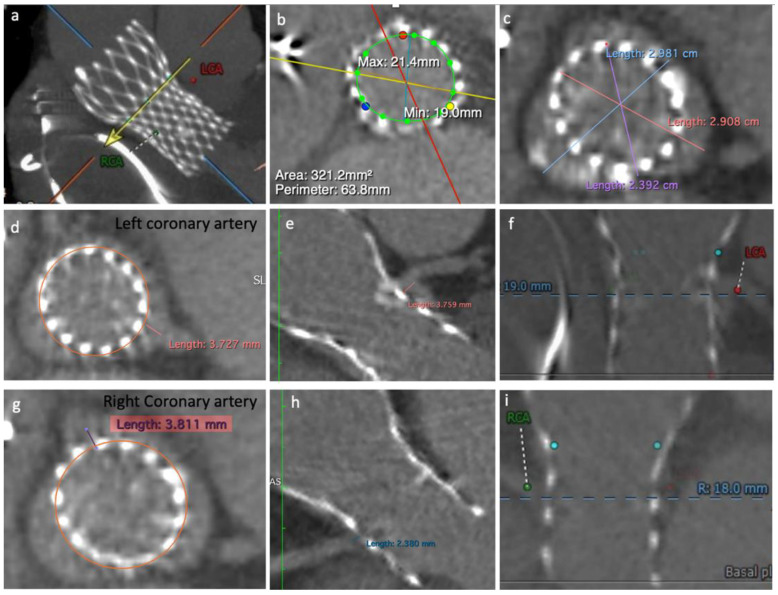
Computed tomography angiography images: (a) CoreValve structure, left coronary artery ostium (LCA) and right coronary artery ostium (RCA); (b) Calculated area and perimeter; (c) Sinus of Valsalva diameter; (d,e) left coronary cusp VTC; (f) LCA height related to the frame bottom; (g,h) right coronary cusp VTC; (i) RCA height related to the frame bottom.
